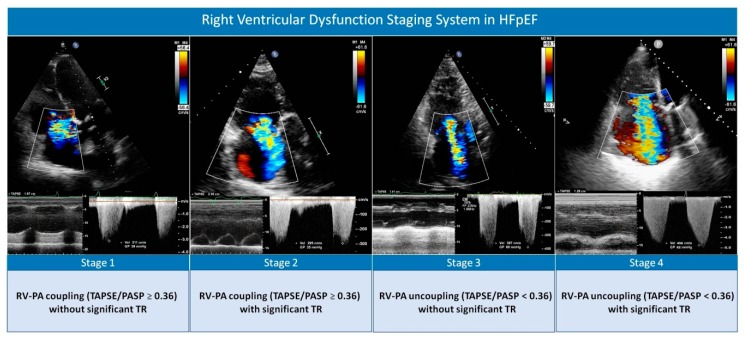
Proposed echocardiographic right heart dysfunction staging classification system based on right ventricular–pulmonary artery coupling and functional tricuspid regurgitation severity. HFpEF: heart failure with preserved ejection fraction; RV–PA: right ventricular–pulmonary artery; TAPSE/PASP: tricuspid annular plane systolic excursion to pulmonary artery systolic pressure ratio; TR: tricuspid regurgitation.
